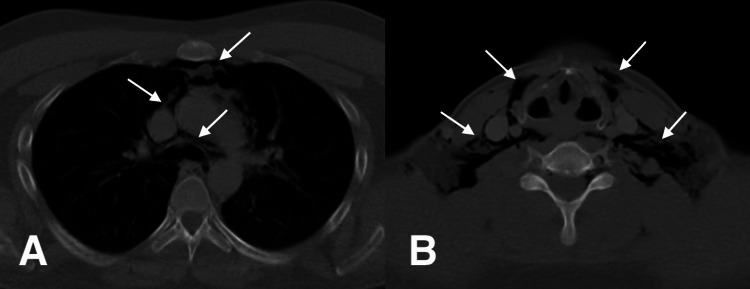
A: Postoperative thirty-sixth-hour, pneumomediastinum detected on thorax CT; B: Postoperative thirty-sixth-hour subcutaneous emphysema detected in the neck region (white arrows)
